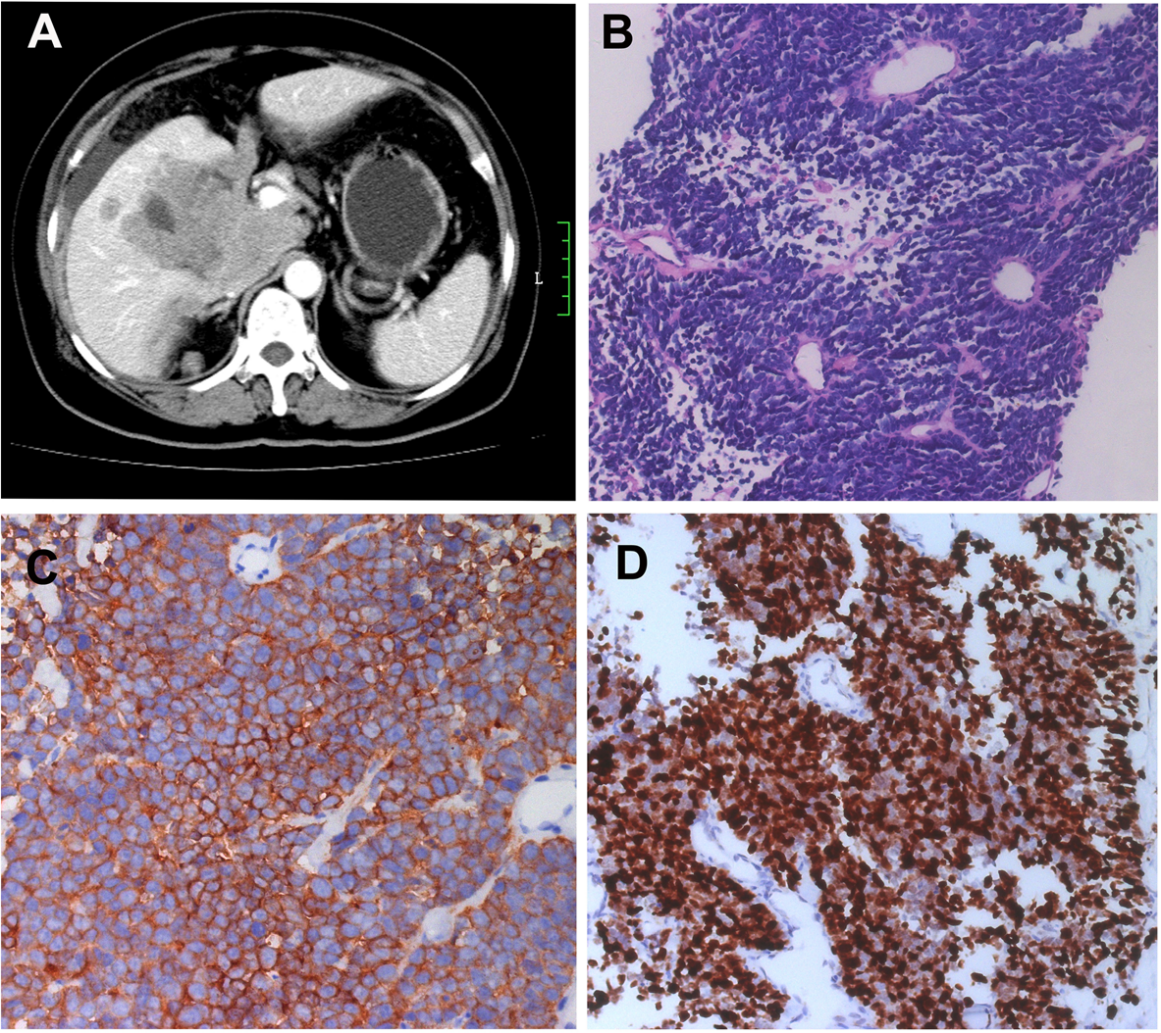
Radiological and pathological data of the case before chemotherapy. A. CT scan showed a gallbladder mass invading liver, head of pancreas with metastasis of peritoneum and enlarged lymphnodes in hepatic hilar. B. Diffuse small round cells with scanty cytoplasm and round nucleus (HE×200). C. Strong positive cytoplasmic expression of Syn (IHC×400). D. Over 80 % tumor cells show positive expression of Ki-67 (IHC×200).
